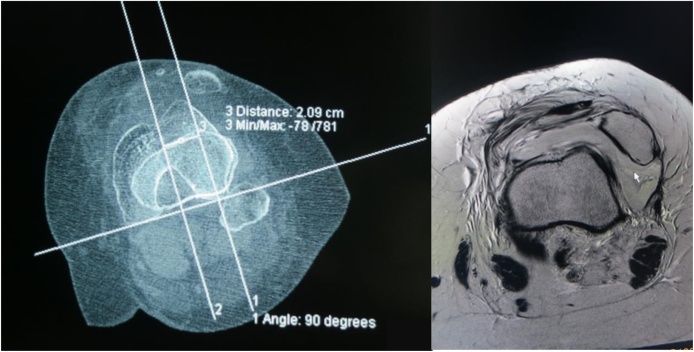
CT scan and MRI show dislocation of patella ‘.
